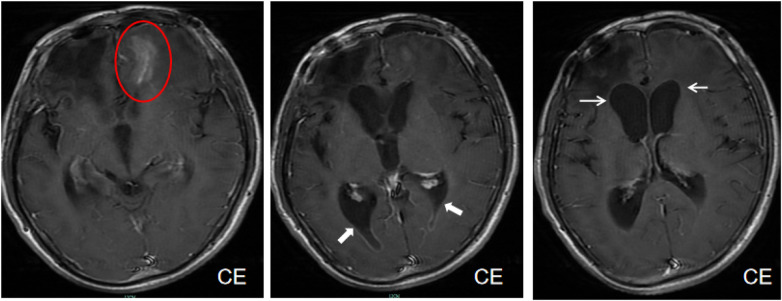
Contrast-enhanced cranial MRI: residual abscess wall is noted (red circle); no empyema (thick arrow)or hydrocephalus (thin arrow) is identified in the cerebral ventricles.
